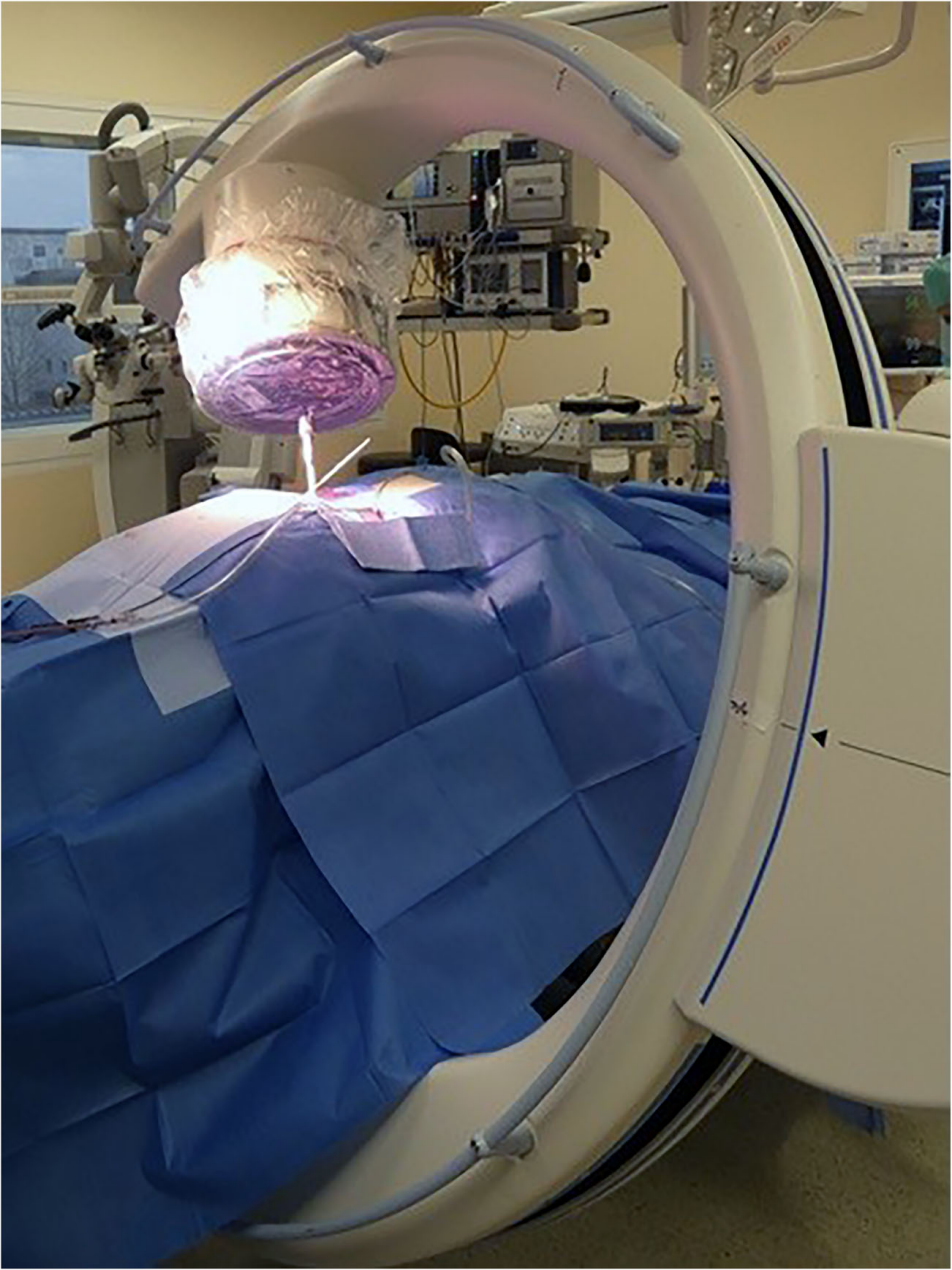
Correct X-ray alignment in 25° sagittal and coronal plane with inserted awl
